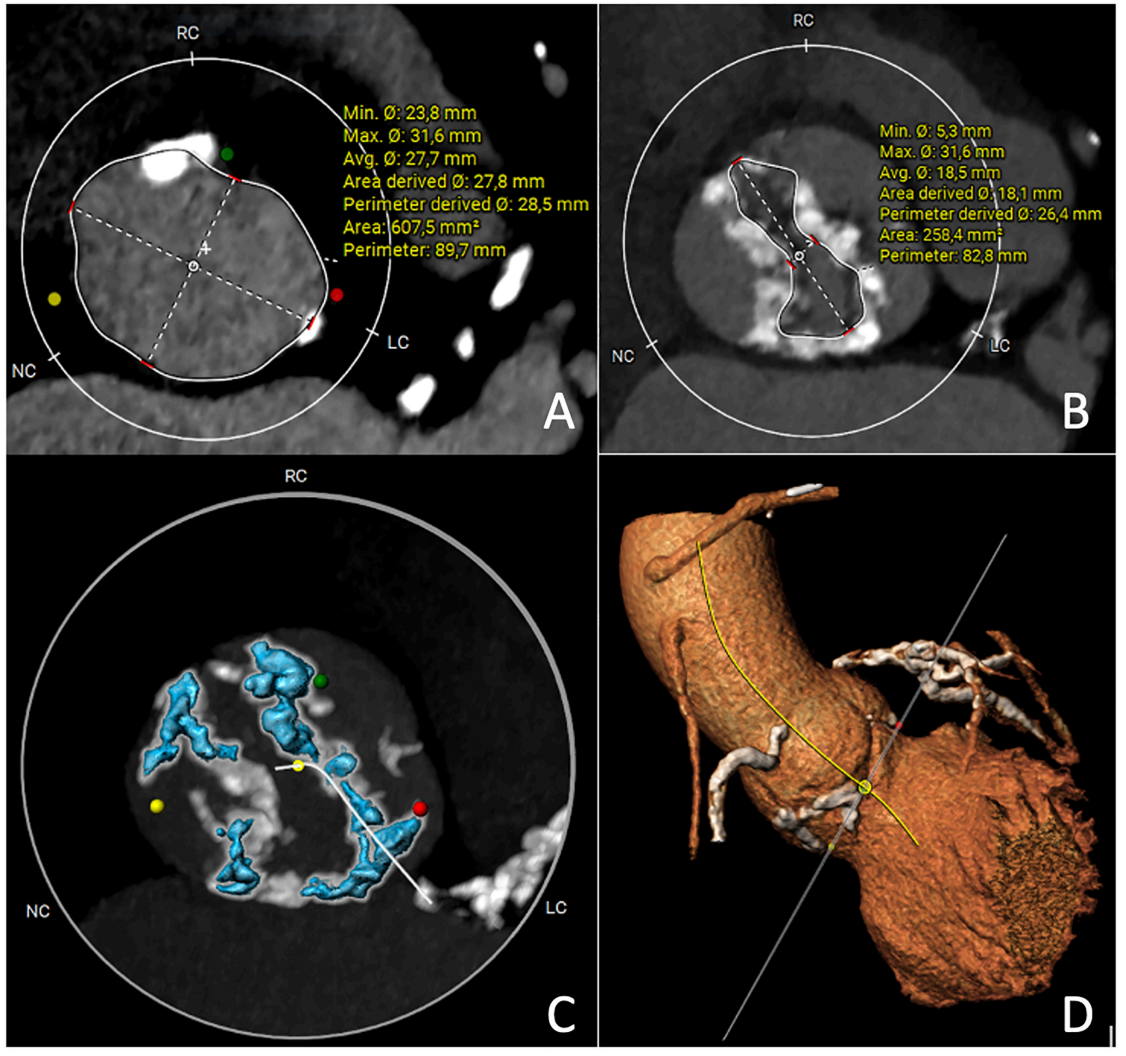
Multislice computed tomography images of bicuspid aortic valve
